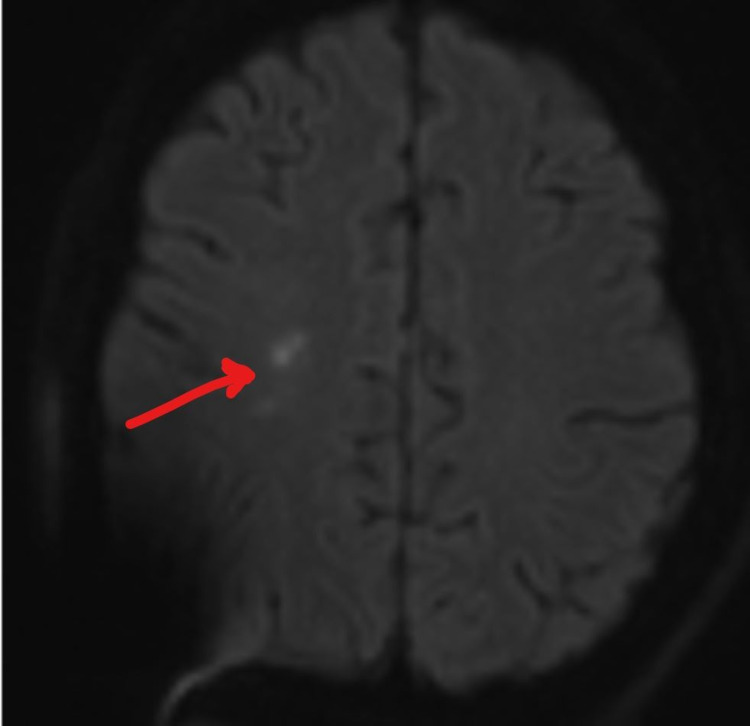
DWI of head MRI, Day 10. DWI: diffusion-weighted imaging.
